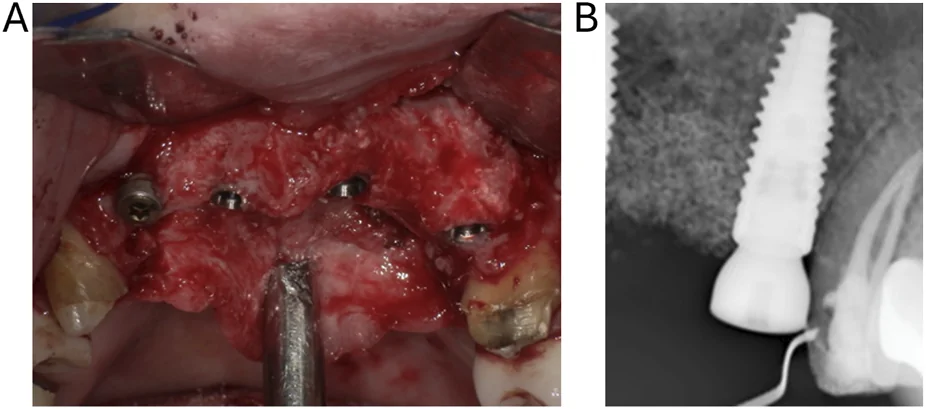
Case 1: 8 months post-grafting. Clinical image (A) showing implant placement into good quality bone. Radiograph shows newly regenerated bone along the full length of the implant (B).
